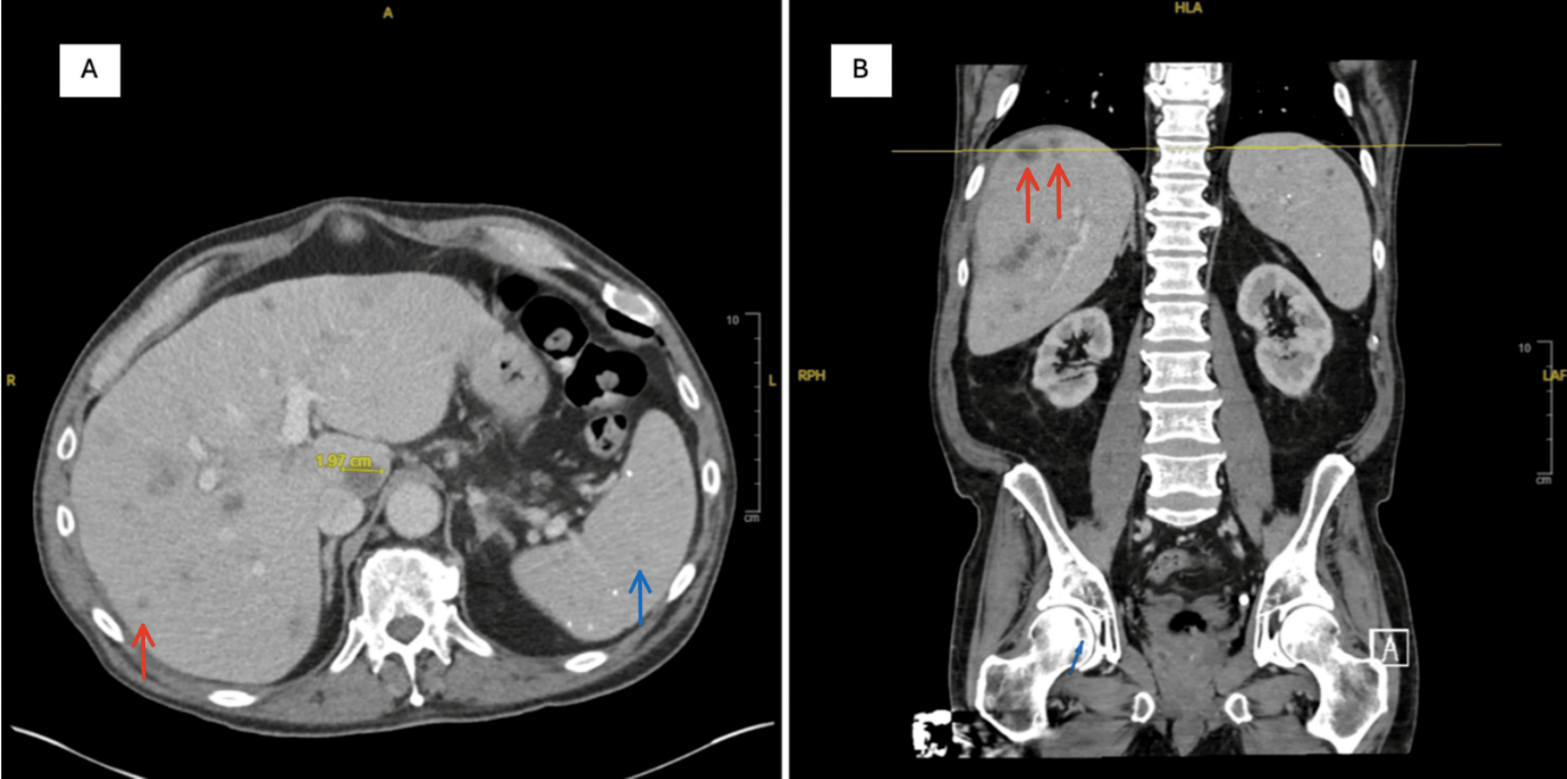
Imaging performed at eight-week follow-up showing improvement of lesions of the liver (red arrows) and the spleen (blue arrow). CT of the abdomen and pelvis with contrast in axial view (A) and coronal view (B).
